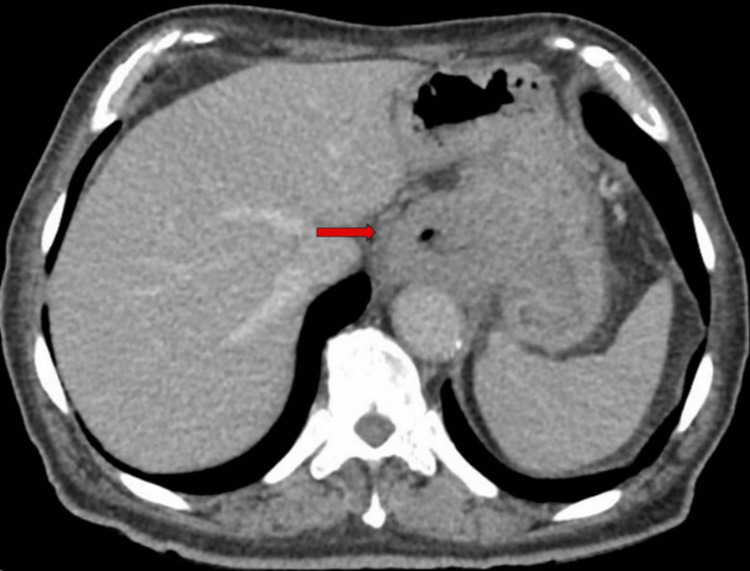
CT image showing primary tumor in the axial plane (red arrow). CT, computed tomography
